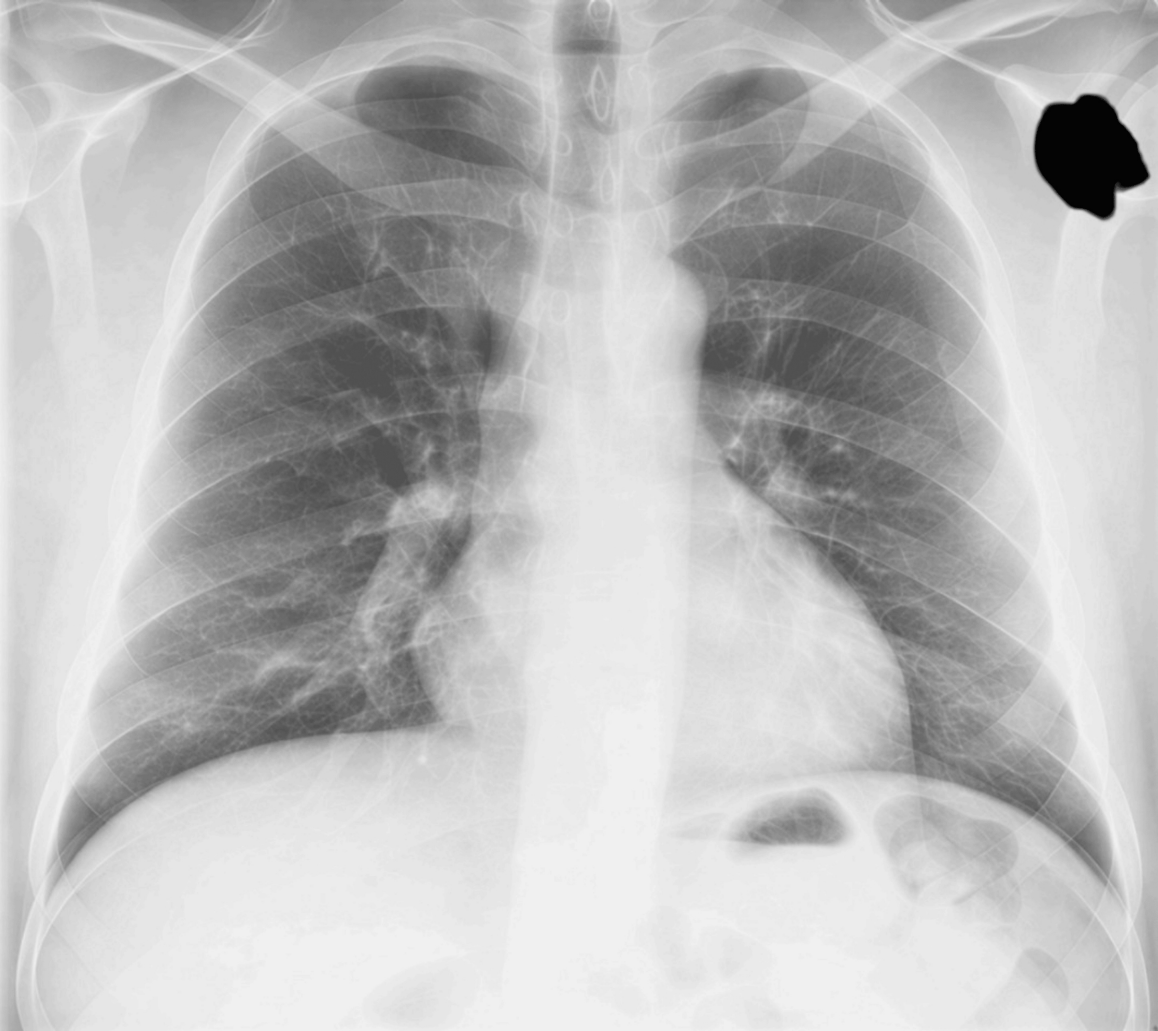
Chest X-ray with normal cardiac silhouette and no features of congestion or consolidation Black mark is used to ensure complete anonymity
